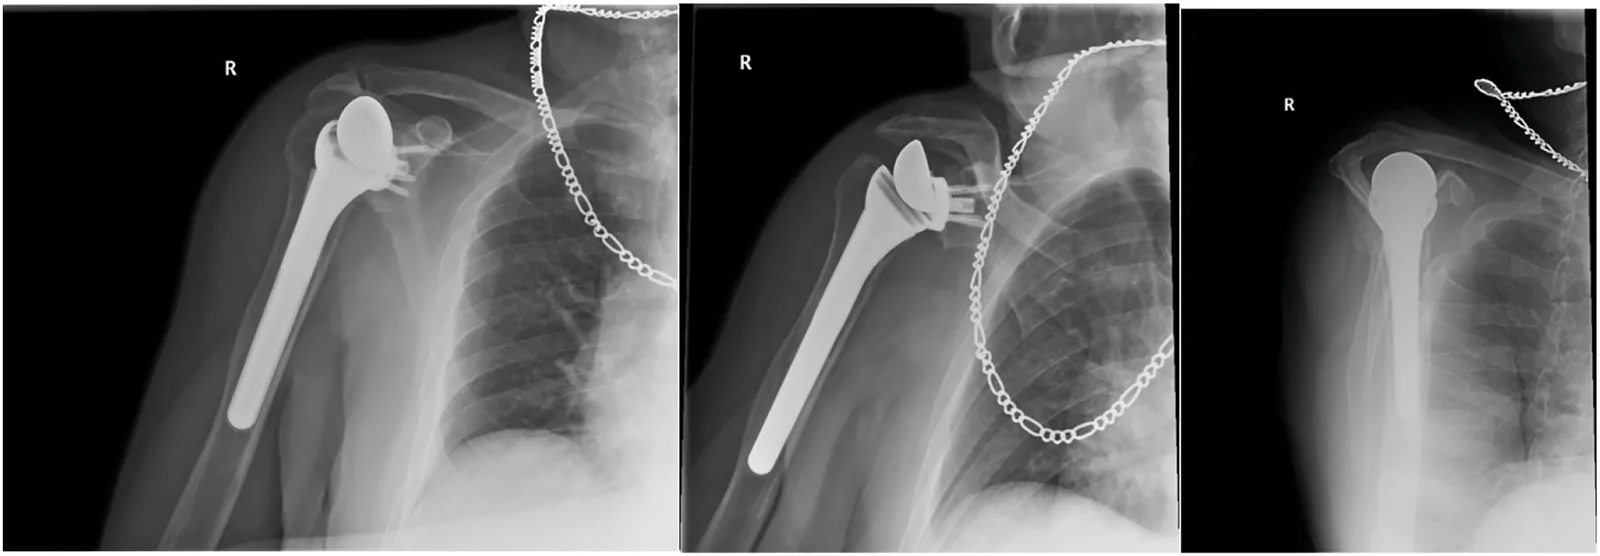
Case 1 x-rays show screw breakage and glenosphere total disengagement.
